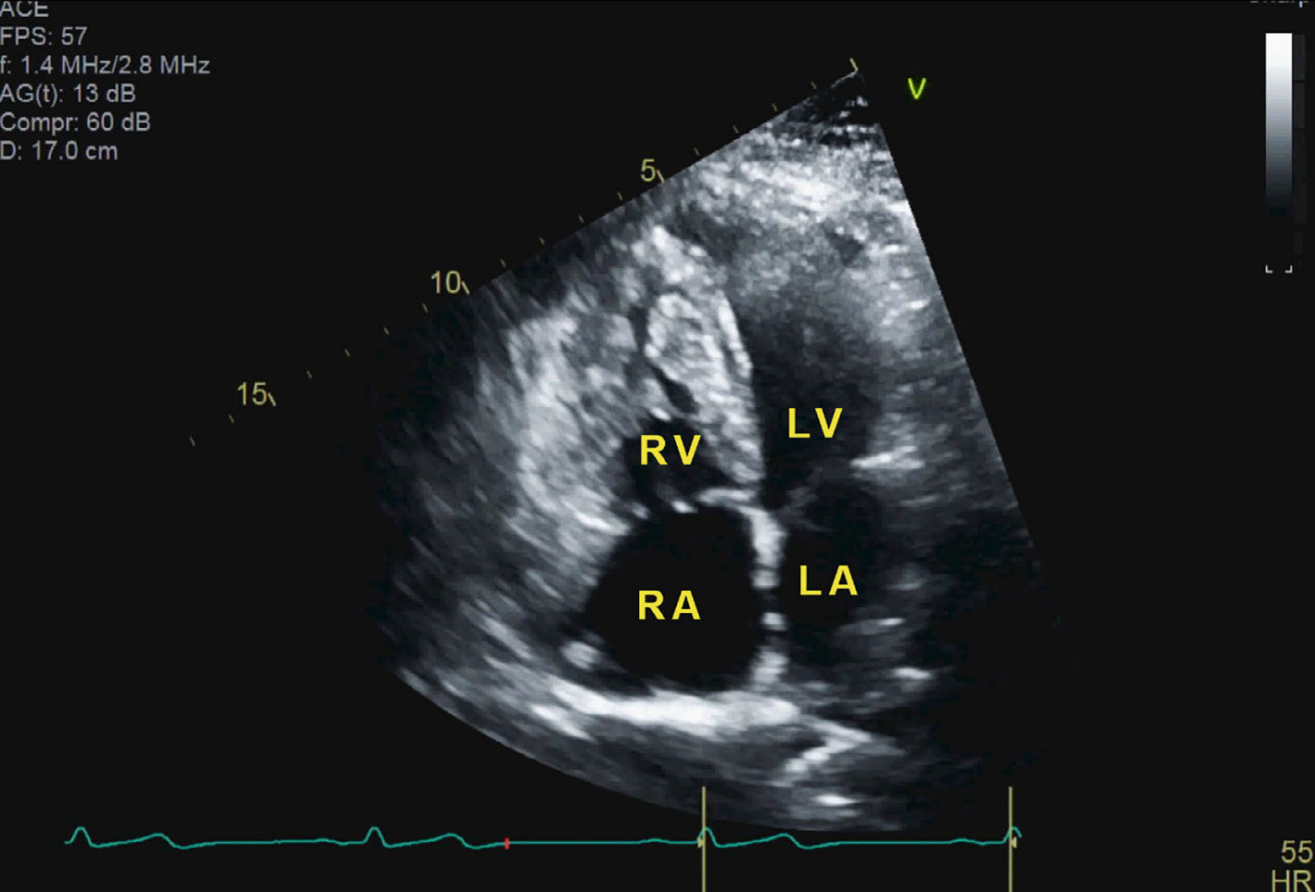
Transthoracic echocardiogram. Four‐chamber view demonstrating right ventricular hypertrophy. Abbreviations: RA, right atrium; RV, right ventricle; LA, left atrium; LV, left ventricle.
